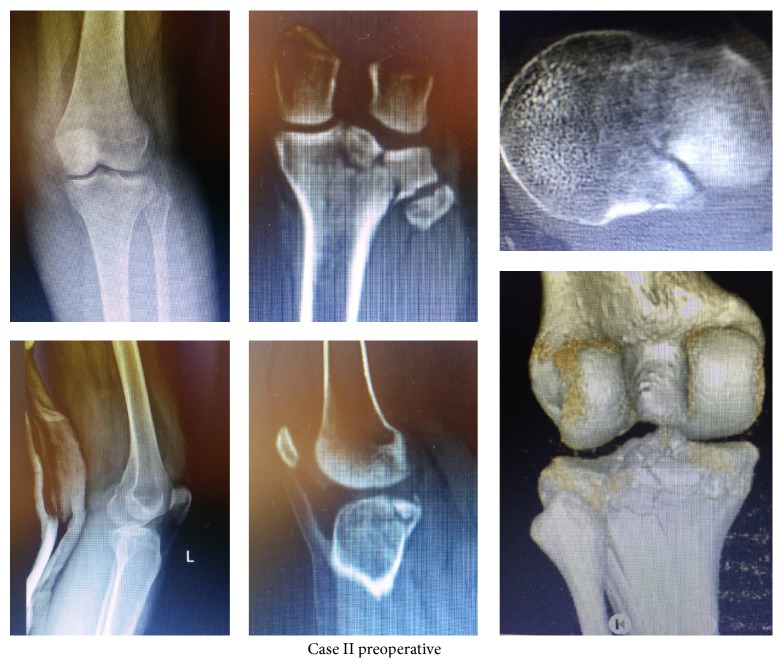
Preoperative radiographies and computed tomography images of Case II.
